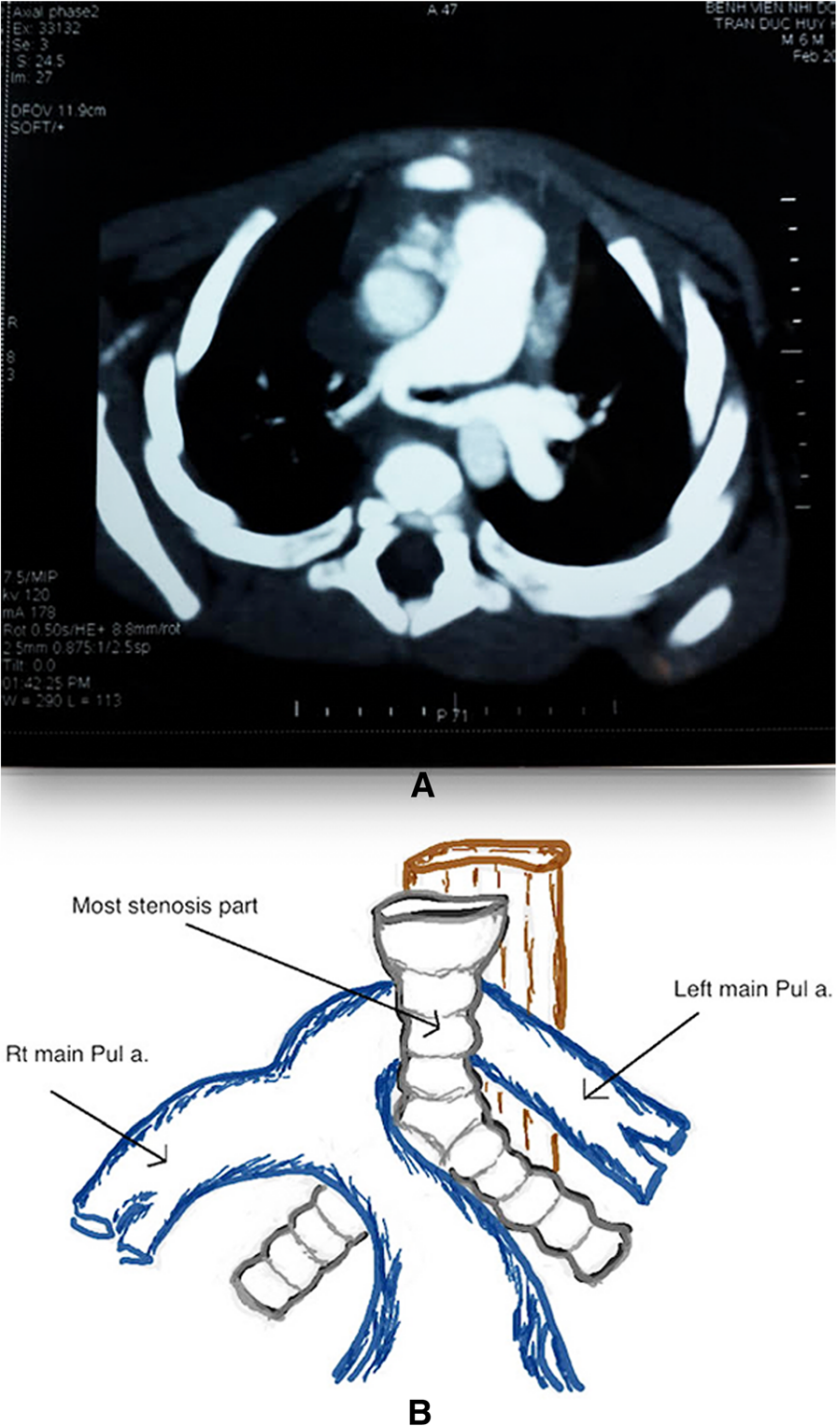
(a and b) CT scan show left main Pulmonary artery sling arrive from the right main Pulmonary artery sling
